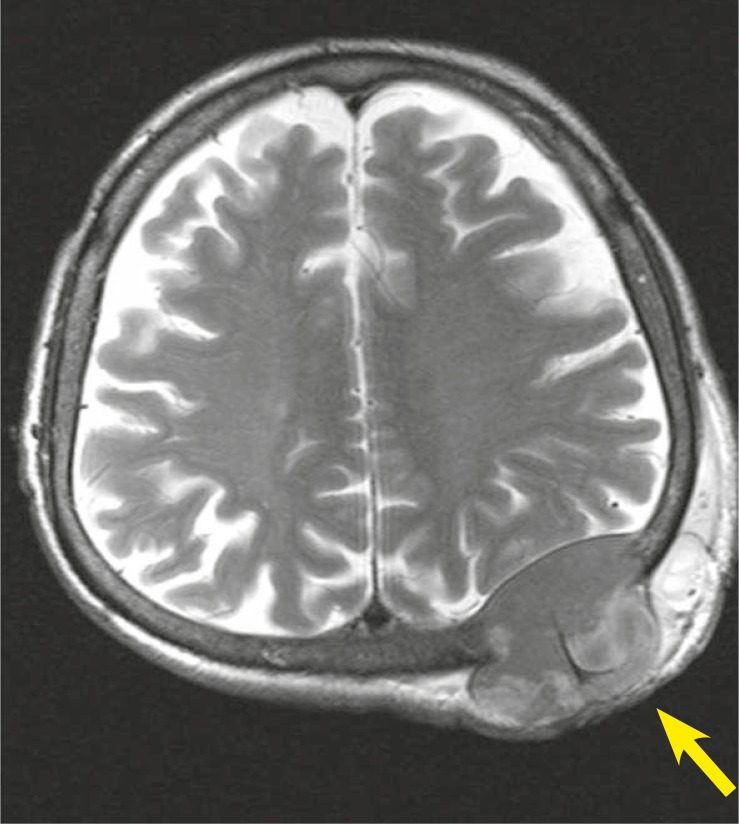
Brain MRI with a subcutaneous metastasis from hepatocellular carcinoma.
